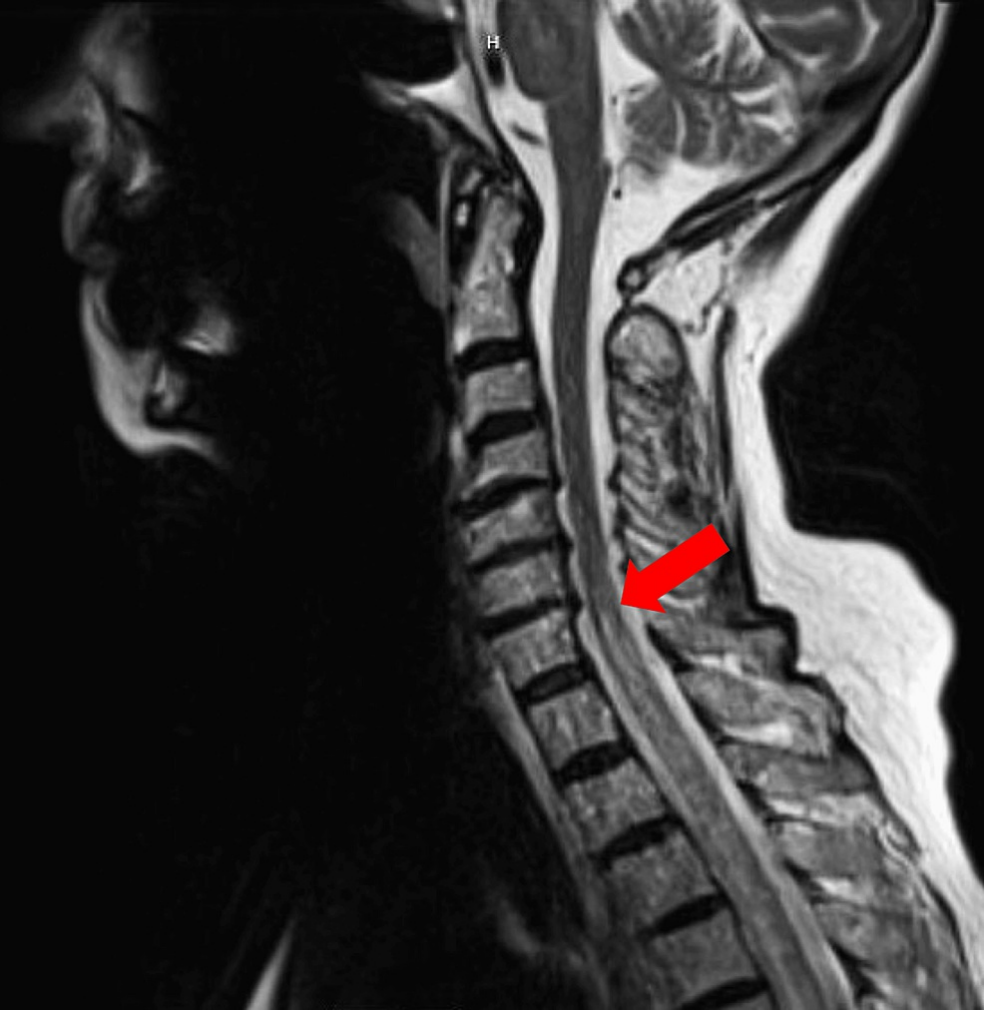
T2-weighted cervical spine MRI, sagittal view, showing increased T2 signal starting at C7 (arrow) MRI: magnetic resonance imaging
